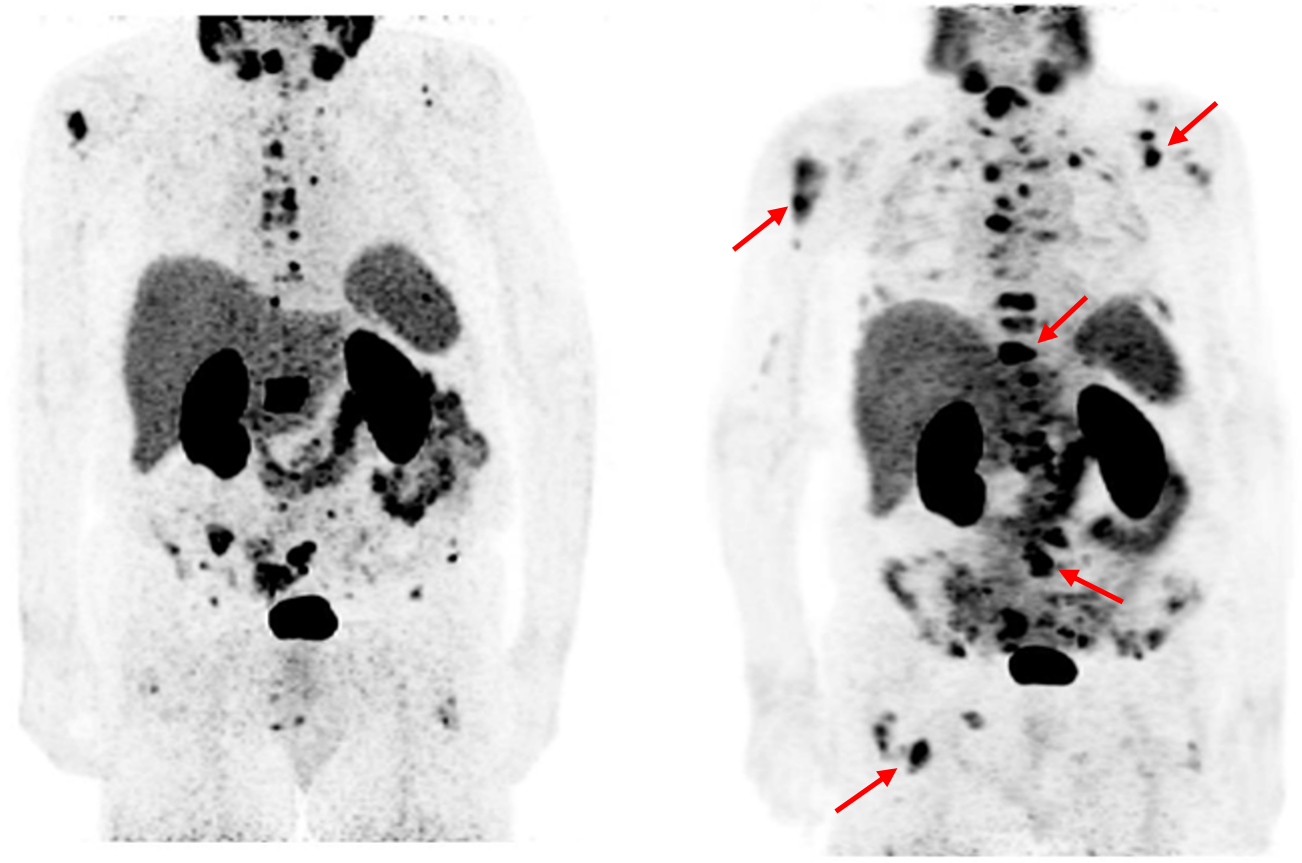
Response evaluation using PMSA PET examinations in patients receiving 3 cycles of [^177^Lu]Lu-PSMA-617 therapies. Among patients not responded to PSMA-RTL, a 78-year mCRPC patient with serum Hb levels below the normal range (11.7 g/dL) and PSA levels of 11.53 μg/L. (right): [^68^Ga]Ga-PSMA-11 PET scan prior PSMA-RLT. (left): [^68^Ga]Ga-PSMA-11 PET scan after obtaining 3 cycles of 7400 MBq PSMA-RLT every 4 weeks, PSA levels increased to 33.46 μg/L and PET scan revealed detection of multiple new PSMA-expressing lesions in bone and lymph nodes (red rows)
